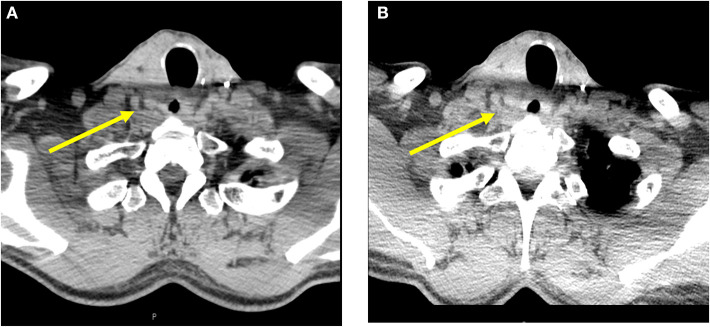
Cross sectional imaging studies with computed tomography of the neck (A) prior to PD-L1 administration and (B) after a 40% decrease in calcitonin, showing stable thyroid bed recurrence.
